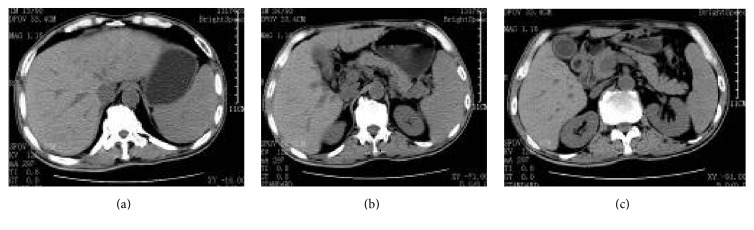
Epigastrium CT after treatment. Note: the liver outline was significantly more prominent. The liver edge was less smooth. Liver parenchymal density was uniform. Multiple quasicircular low-density foci were found in the right lobe of the liver, but no intensified low-density focus was noted. The largest diameter was about 5.5 mm. The spleen was enlarged to approximately seven rib units. The density was uniform. Compared with the CT findings on July 11, the liver density increased and the density was more uniform.
